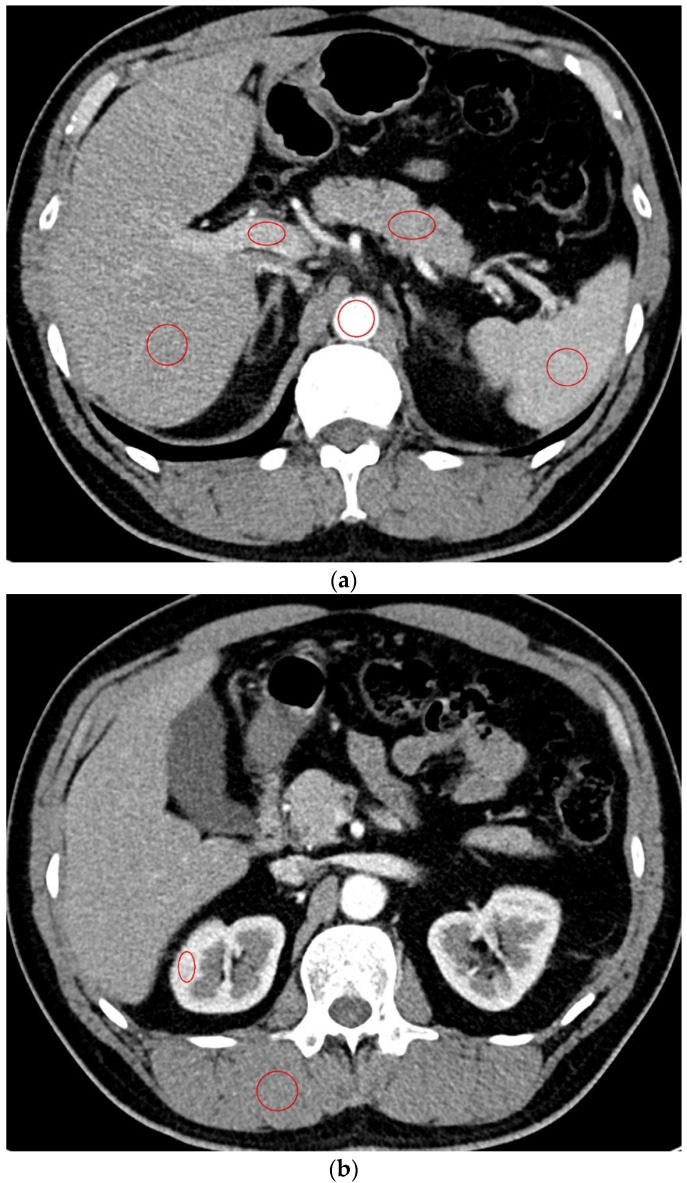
Quantitative analysis of the CT images A radiologist draws multiple regions of interest in the liver, pancreas, spleen, portal vein, and aorta (a) and in the kidney and paraspinal muscle (b).
